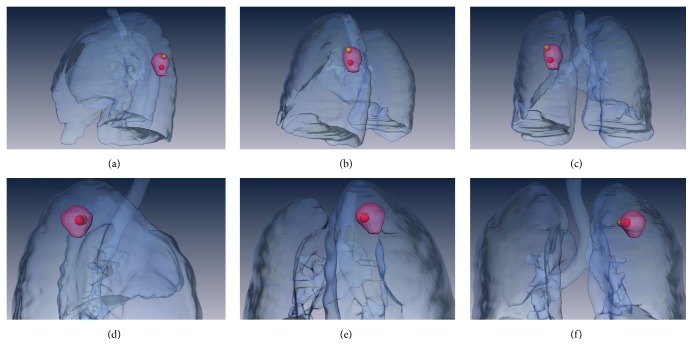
3D visualization of patient cases with spatial mismatch and match between ADC_min_ and SUV_max_. (a–c) Patient with adenocarcinoma in the left lower lobe superior segment in lateral (a), posterior oblique (b), and posterior (c) projections. (d–f) Patient with adenocarcinoma in the right upper lobe apical segment in lateral (a), posterior oblique (b), and posterior (c) projections. The orange points indicate the ADC_min_ and the red points the SUV_max_. The distance between both parameters in the first patient is 19.5 mm and 5.3 mm in the second patient.
